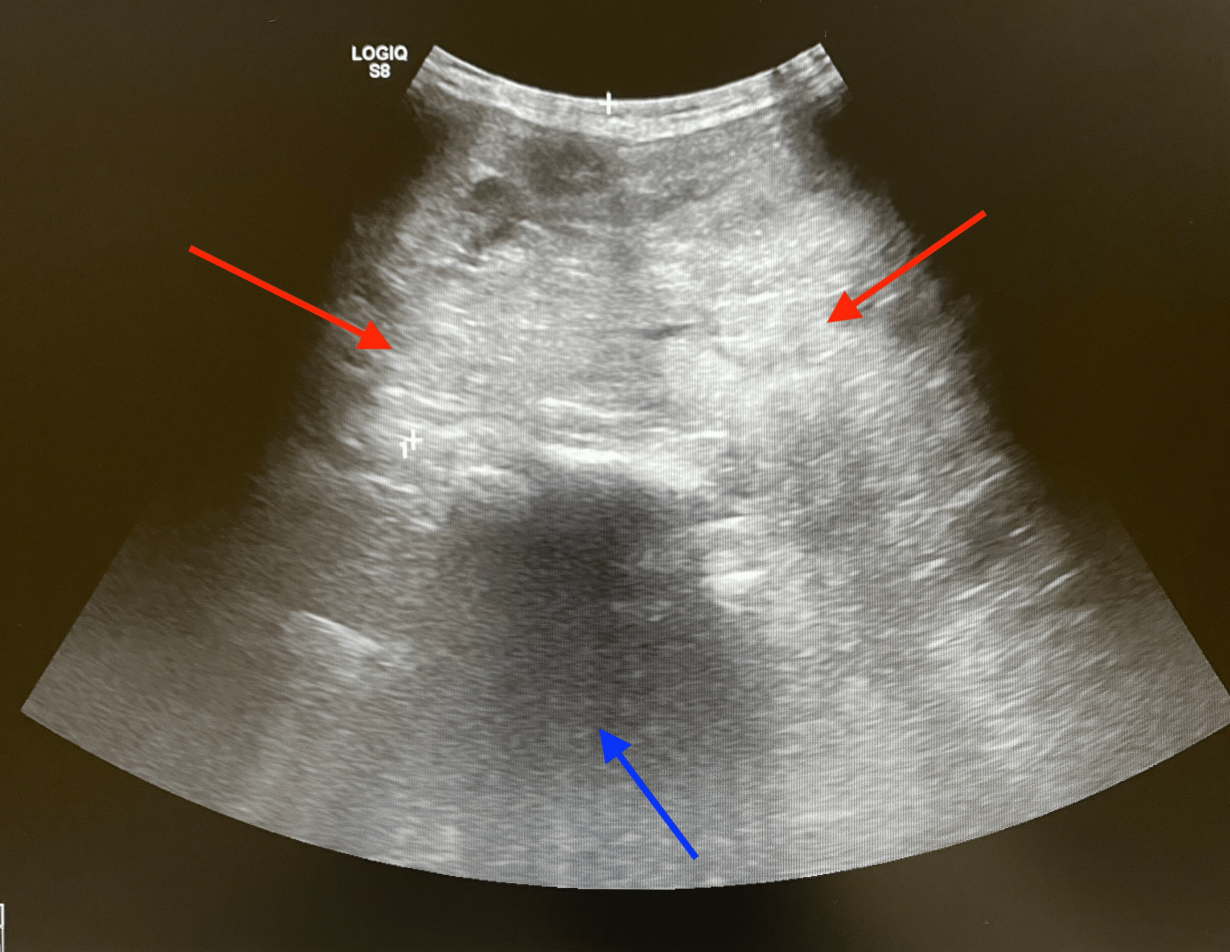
Ultrasound of the neck in the transverse plane showing markedly enlarged and heterogeneous thyroid tissue consistent with goiter (red arrows). The hypo-echoic trachea is displaced leftward by the enlarged right thyroid lobe (blue arrow).
